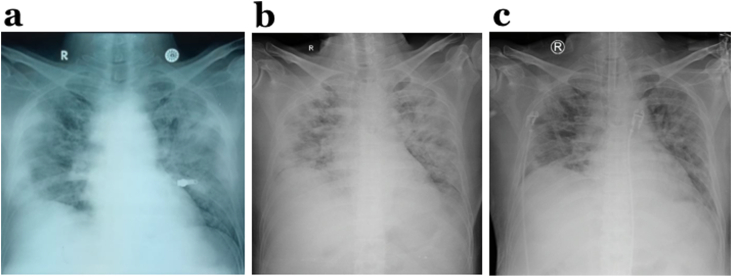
Chest x-rays: a) on the admission day indicated bilateral pneumonia, which is not compatible with the relatively slight clinical manifestations of patient, b) on the third day, and c) on the eight day also showed bilateral pneumonia.
